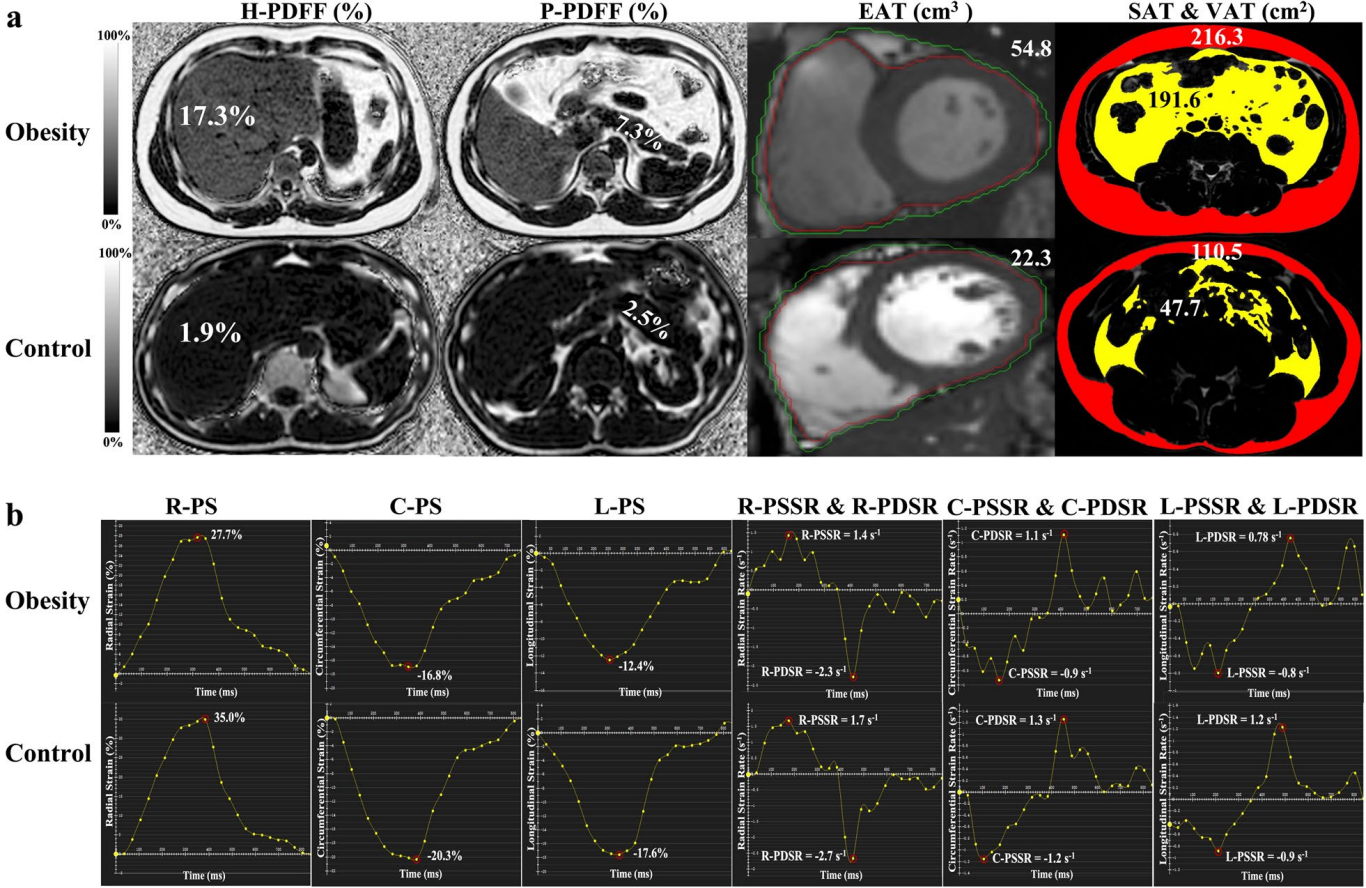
Examples of (a) MR images and (b) LV myocardial strain parameters in a 39-year-old man with obesity and in a healthy 34-year-old man
